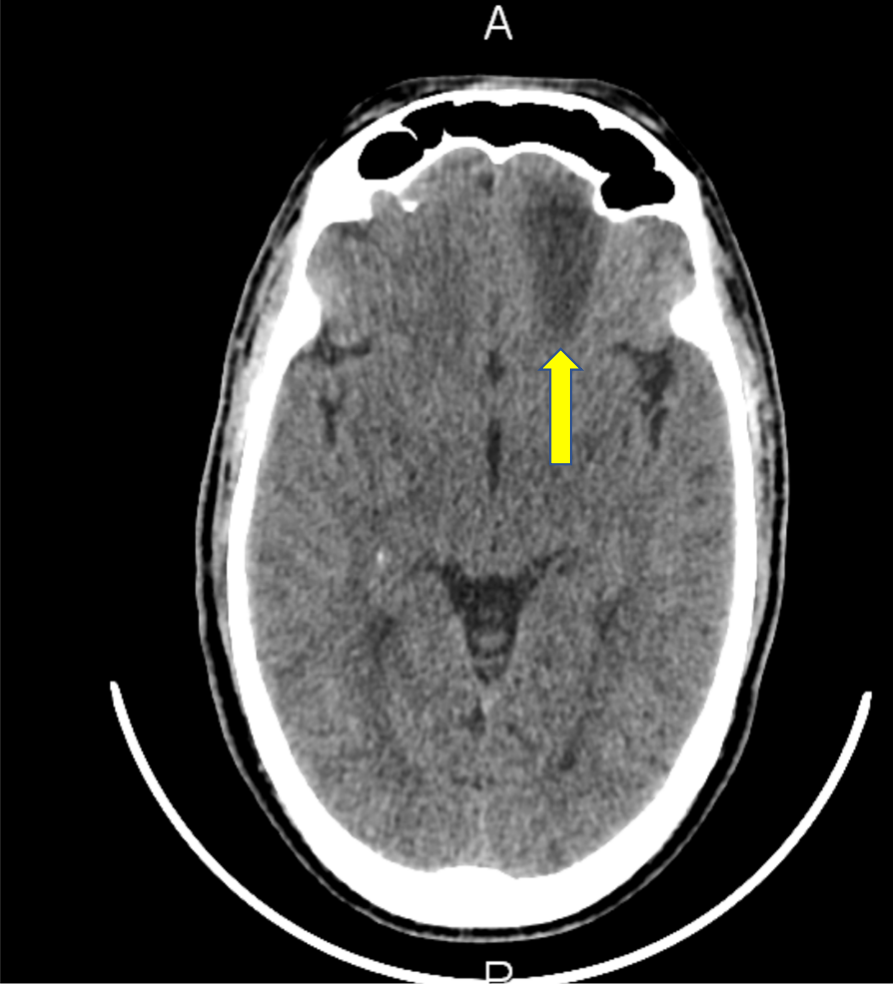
Initial non-contrast CT scan of the brain revealing a hypodensity in the left frontal region of the cerebrum
